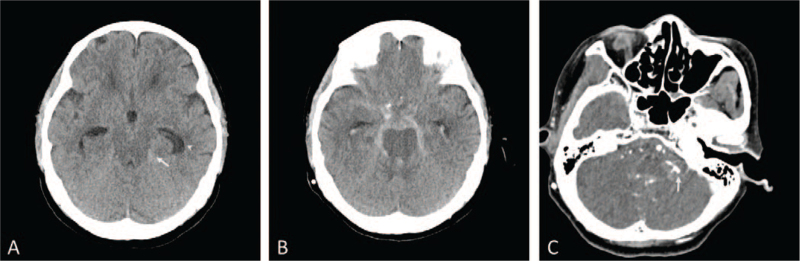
(A) Initial non-contrast brain computed tomography (CT) scan showed a scanty subarachnoid hemorrhage (SAH) on left ambient cistern (arrow) with enlarged ventricle (arrow head). (B) The brain CT taken at 10th hospital day revealed SAH on all cistern with intraventricular hemorrhage. (C) The thin axial source images of CT angiography taken at 10th hospital day showed a suspicious lesion of aneurysm on distal posterior inferior cerebellar artery (arrow).
